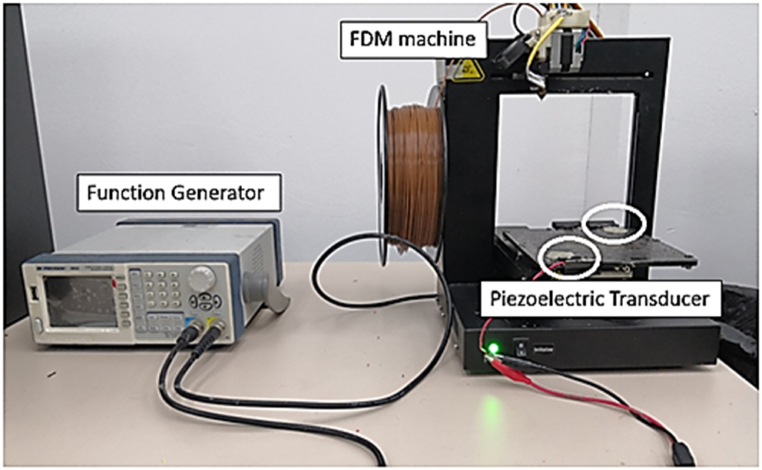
Experimental set-up of ultrasonic-assisted FDM system.
